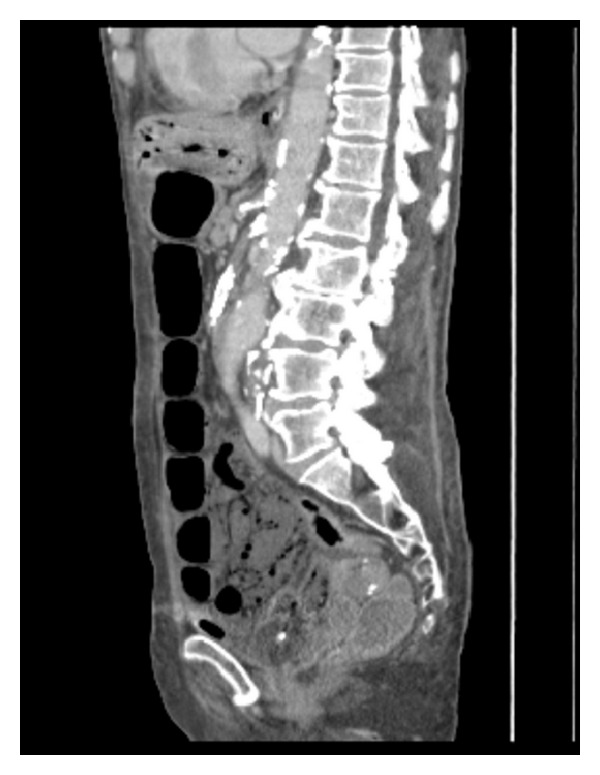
CT showing nonenhancement of the superior mesenteric artery following contrast. The celiac axis is heavily calcified with minimal contrast enhancement (Case 2).
